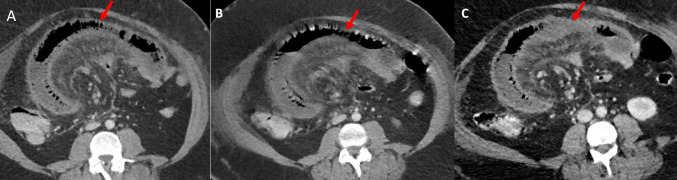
CT examinations at day 2 (A), day 4 (B), and day 6 (C) post presentation showing marked ischaemic change within a loop of jejunum (solid arrow)
